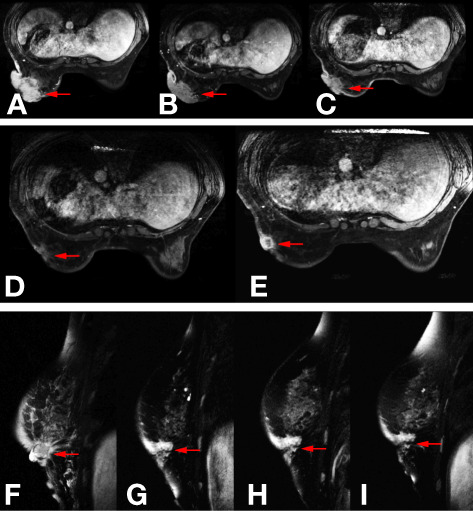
Images of mammary magnetic resonance imaging (MRI) in different phases. (A) The baseline mammary magnetic resonance imaging (MRI) in November 2017. (B) Mammary MRI after five cycles of TCbH regimen in February 2018. (C) Mammary MRI after four cycles of ECH regimen in May 2018. (D) Mammary MRI after two cycles of nab-paclitaxel plus trastuzumab regimen in July 2018. (E, F) Mammary MRI after four cycles of nab-paclitaxel plus trastuzumab regimen in August 2018. (G) Mammary MRI after two cycles of pyrotinib plus capecitabin regimen in October 2018. (H) Mammary MRI after four cycles of pyrotinib plus capecitabin regimen in November 2018. (I) Mammary MRI after six cycles of pyrotinib plus capecitabin regimen in December 2018.
